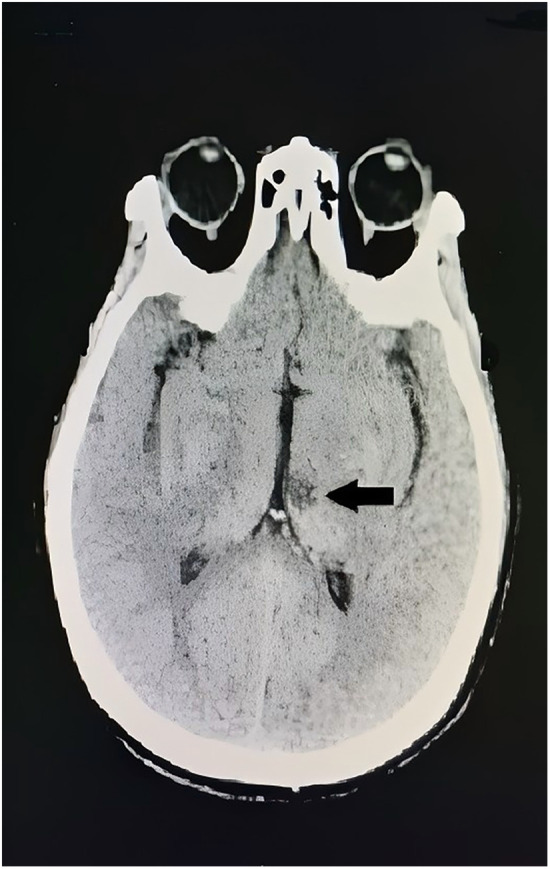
Follow-up head computed tomography scan performed 24 h after stroke onset showing a faint hypodense lesion in the left thalamus (black arrow).
